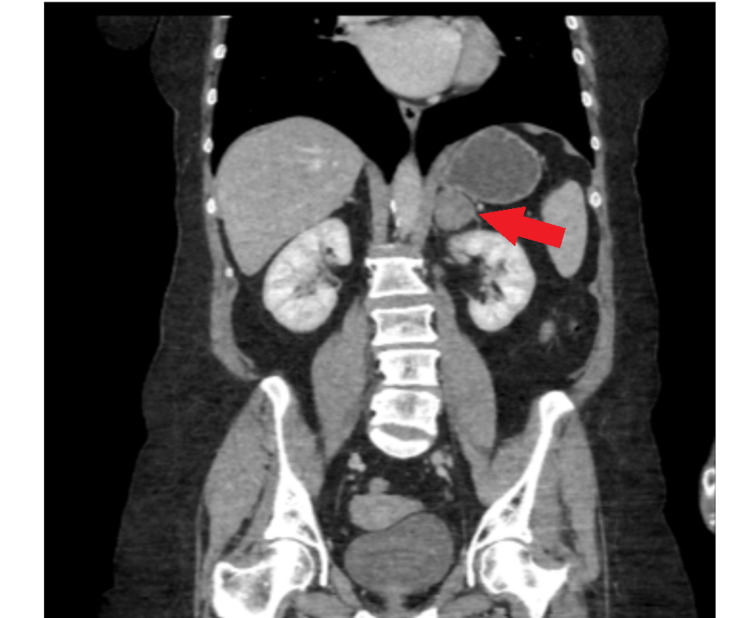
Computed tomography (CT) of the abdomen showing the left adrenal mass
